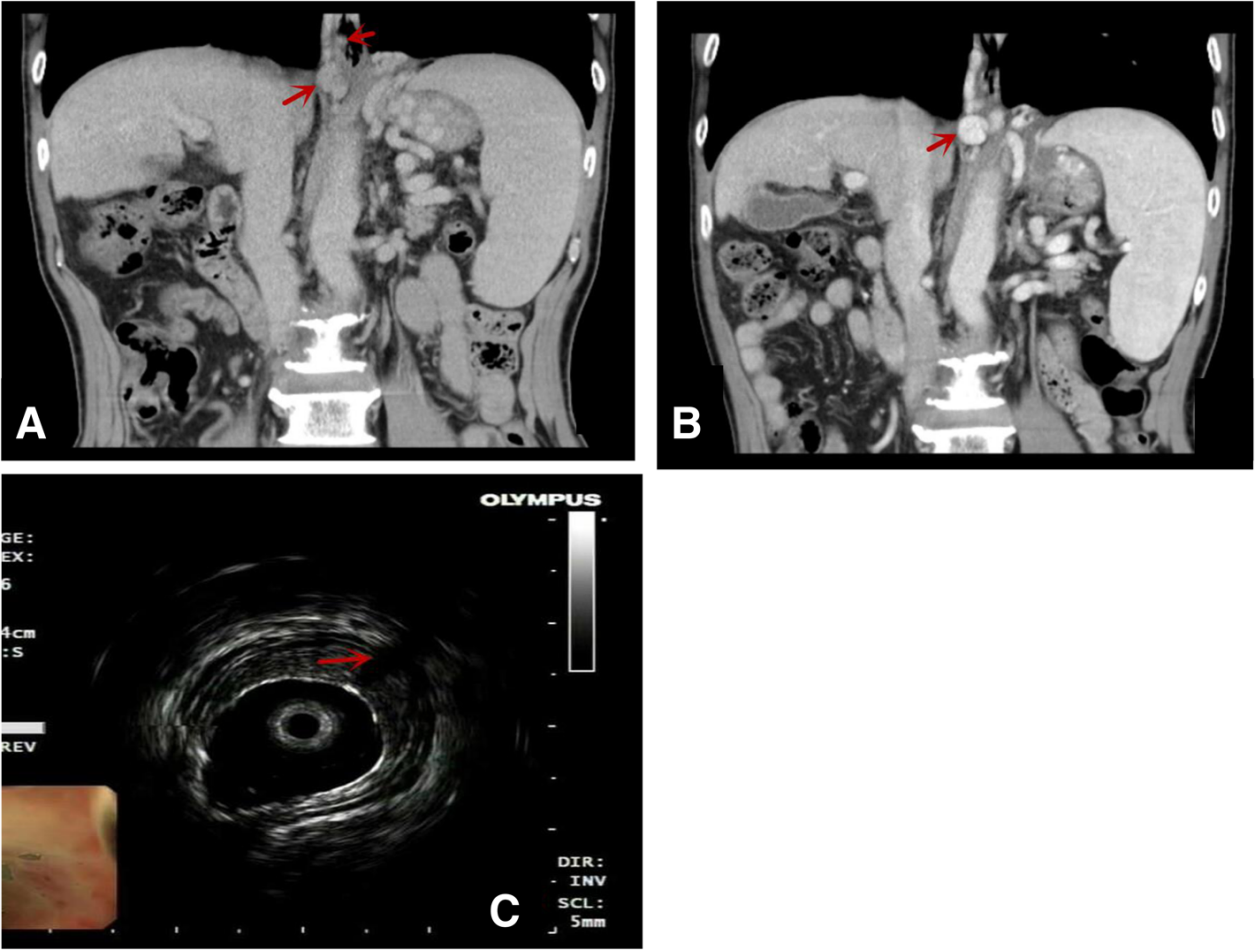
Comparison of CT vascular images before and after endoscopic treatment. a: Before the endoscopic treatment, varicose veins and esophageal varices near the esophagus can be seen. b: After endoscopic treatment, esophageal varices disappear, and varicose veins around the esophagus still exist. It is not well detected the perforating veins (PFV),but EUS can be shown(c:arrow)
